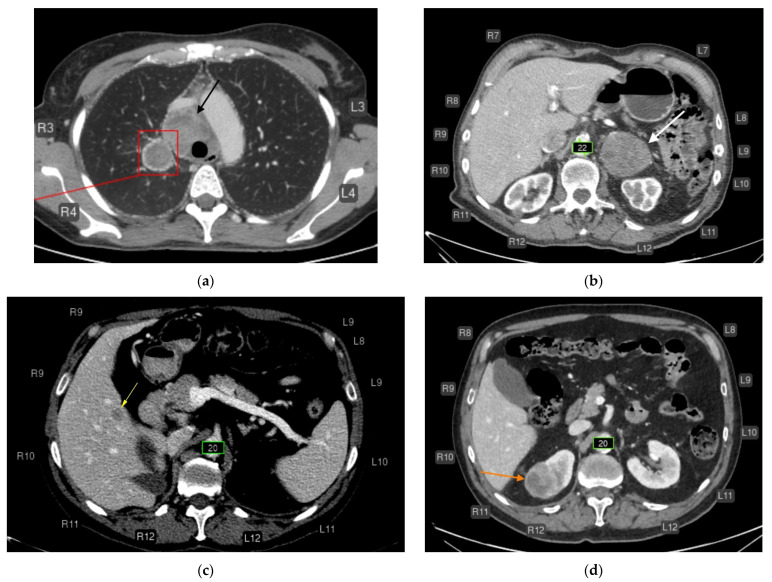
CT of the chest (a) and abdomen (b–d) after AI processing. Clinically significant findings missed by the AI that could substantially affect patient outcomes (false-negative errors). No segmentation (missed pathology): (a) Enlarged intrathoracic lymph nodes (black arrow), possibly representing metastases. False-negative error by the AI service. In addition, the AI correctly labeled the pulmonary lesion and rib numbering. (b) Left adrenal mass (white arrow), possibly a tumor or metastasis. False-negative error by the AI service. The AI correctly measured the abdominal aortic diameter and labeled rib numbering. (c) Hypovascular liver mass (yellow arrow), possibly a metastasis. False-negative error by the AI service. The AI correctly measured the abdominal aortic diameter and labeled rib numbering. (d) Right renal mass (orange arrow), possibly a tumor. False-negative error by the AI service. The AI correctly measured the abdominal aortic diameter and labeled rib numbering.
